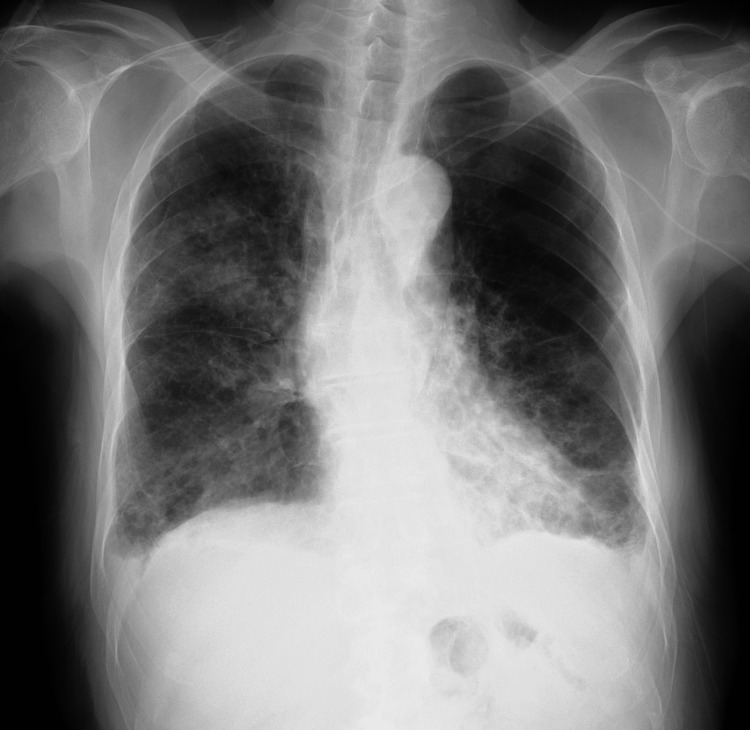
Chest radiograph obtained during postoperative pneumonia after total gastrectomy. The patient developed pneumonia complicated by respiratory failure with CO₂ narcosis, which improved with antibiotic therapy. Considering this recent respiratory complication and underlying severe emphysema, inguinal hernia repair was subsequently performed under local anesthesia with multimodal anesthesia rather than general anesthesia.
